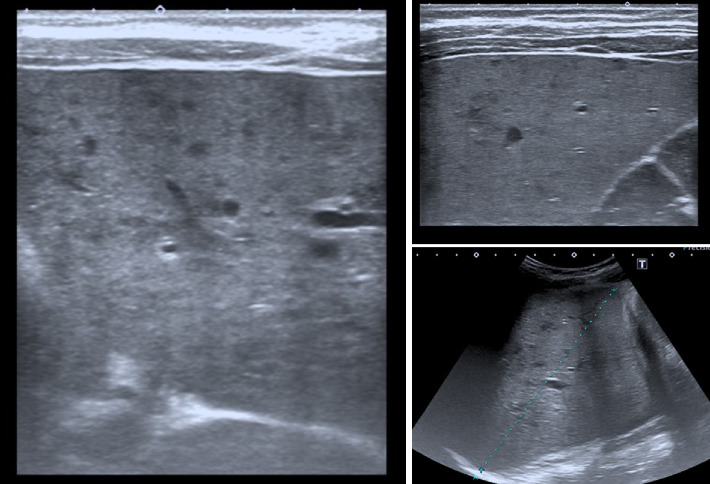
Abdominal ultrasound. This ultrasound shows hepatomegaly with scattered micronodular liver infiltration.
